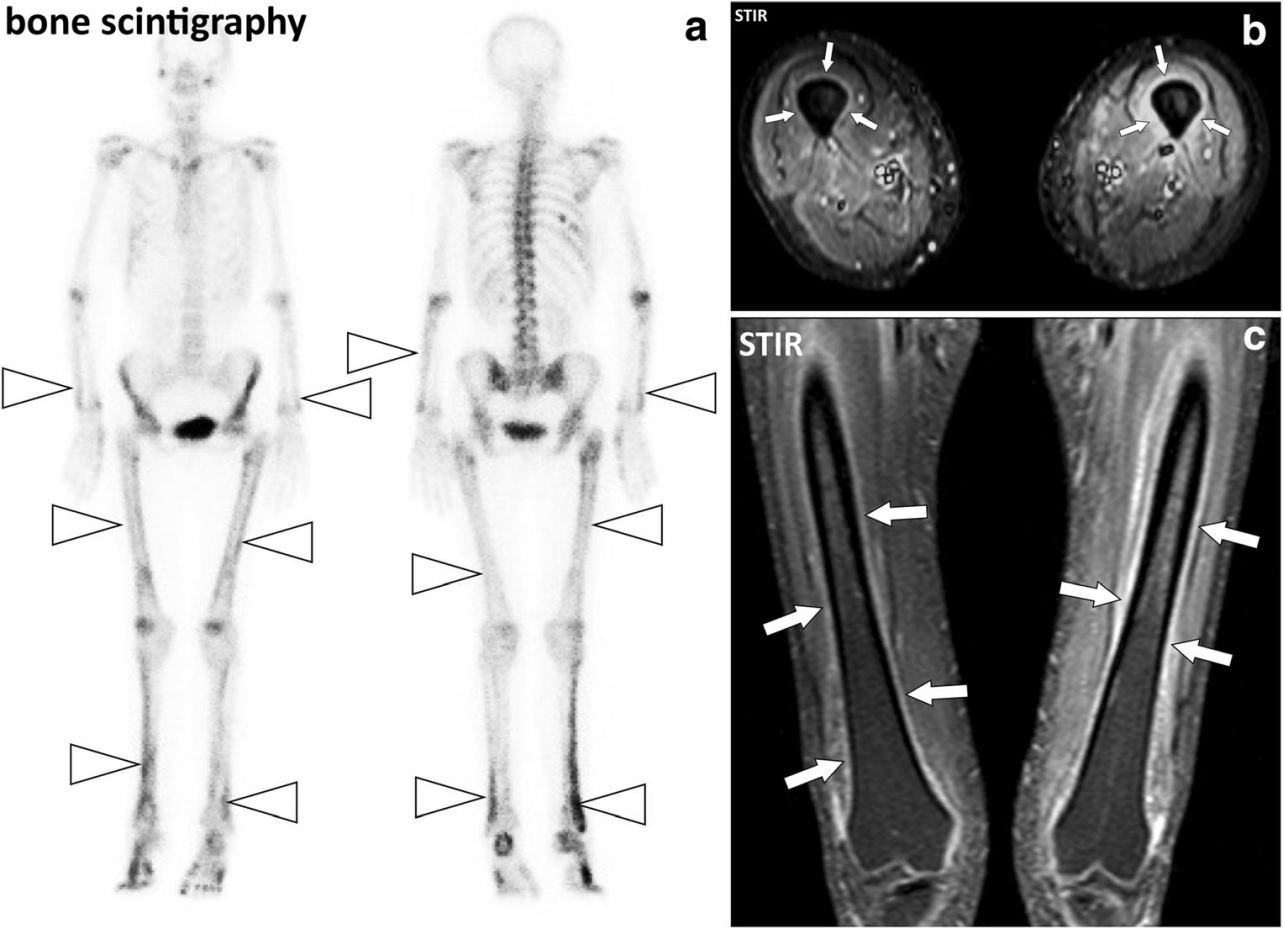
a Anterior and posterior whole-body bone scintigraphy, (b) axial and (c) coronal STIR images of the thighs of a 55-year-old female with pulmonary neoplasm and hypertrophic osteoarthropathy. Bone scintigraphy demonstrates symmetric linear increased uptake of tracer along the diaphysis and metaphysis of the long bones (arrowheads). MRI confirms periostitis with thickening and fluid signal intensity of the periosteum of the left femur and to a lesser degree of the right femur (arrows)
